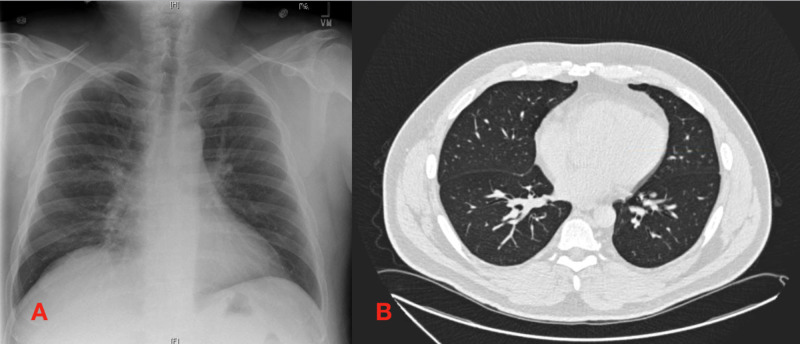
Final chest X-ray on discharge and CT at follow-up A: chest X-ray on the day of discharge demonstrates mild residual right middle lobe opacity; B: follow-up chest CT six weeks after discharge demonstrates resolution of previous bilateral ground-glass and nodular opacifications, with no evidence of residual disease CT: computed tomography
